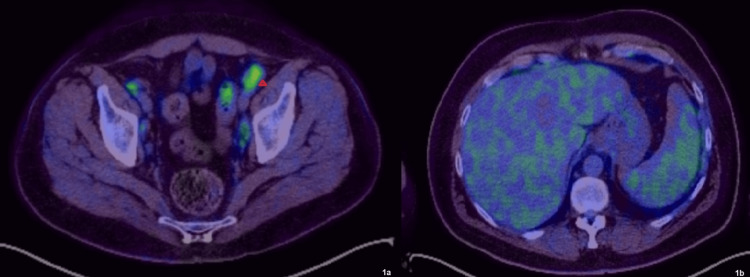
Axial views of FDG-PET/CT. (a) PET/CT demonstrating moderately avid left external iliac lymph node (SUV = 7.0) shown with red arrow. (b) PET/CT demonstrating mild splenic enlargement, which was marginally more active than the liver (SUV max = 4.2 vs. SUV max = 3.7). PET: positron emission tomography; FDG: fludeoxyglucose; CT: computed tomography; SUV: standardized uptake value.
